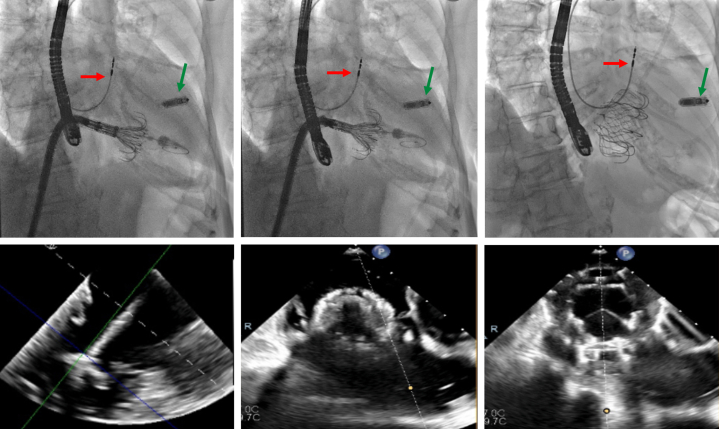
Transcatheter Tricuspid Valve Replacement Steps Fluoroscopic (upper panel) and equivalent echocardiographic (lower panel) steps for orthotopic transcatheter tricuspid valve replacement with the Evoque system. The red arrow indicates the remaining right atrial lead, the green arrow indicates the leadless right ventricular pacemaker.
